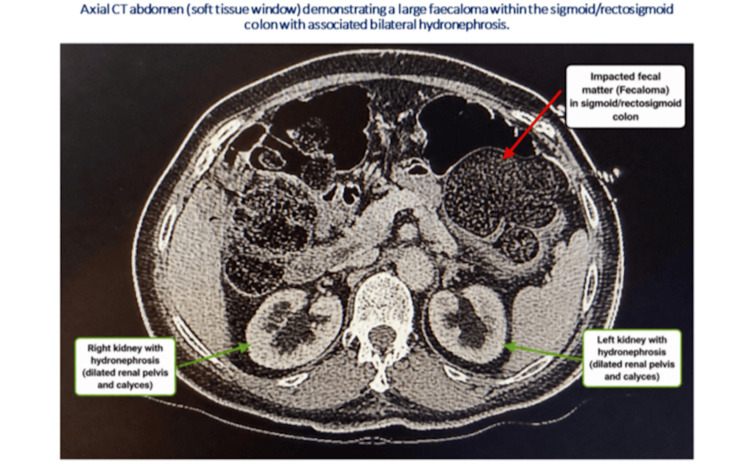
Contrast-enhanced CT of the abdomen (axial, soft-tissue window). Axial contrast-enhanced CT of the abdomen (soft-tissue window) demonstrating marked fecal loading with a large fecaloma within the sigmoid/rectosigmoid colon measuring approximately 7.1 × 6.2 cm, consistent with fecal impaction (red arrow). Associated bilateral hydronephrosis is present, evidenced by dilatation of the renal pelvis and calyces (green arrows) with renal pelvic dilatation measuring approximately 2.0 cm on the right and 1.9 cm on the left. Findings are in keeping with stercoral colitis causing extrinsic compression of the urinary bladder and distal ureters and resultant obstructive uropathy.
